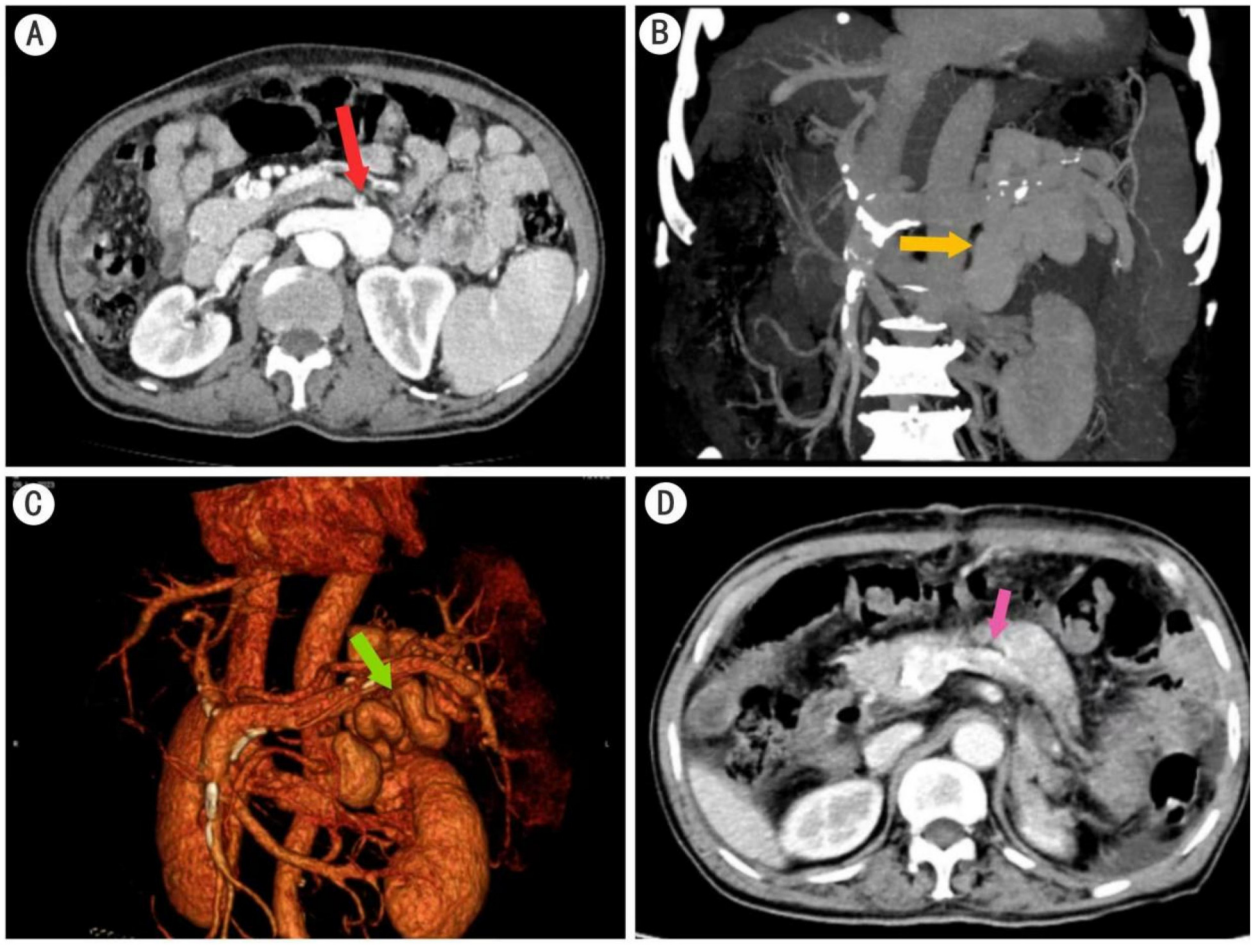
320-slice CT portal vein imaging. (A) The coronal view demonstrates enlargement of the splenorenal shunt. (B,C) Sagittal views reveal the thickened and tortuous splenorenal shunt. (D) Postoperative image shows the absence of the spleen and narrowing of the previously dilated portal vein with complete obliteration of the splenorenal shunt.
